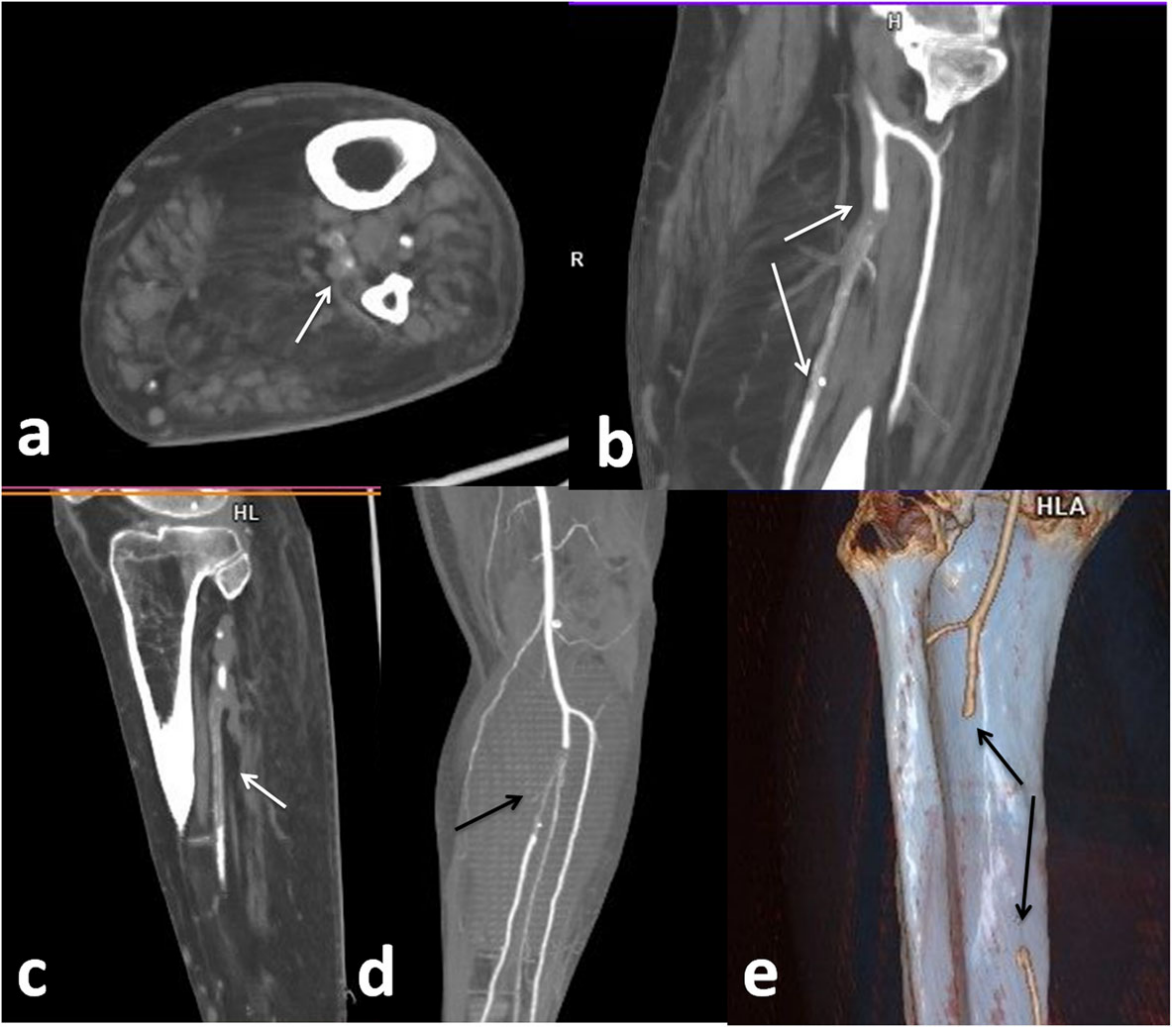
Female patient 52 years old. Acute thormbus of proximal seep peroneal artery (white arrows in a,b,c), CTA (black arrow in d), 3D reconstructed image (filling defect black arrows in e)
